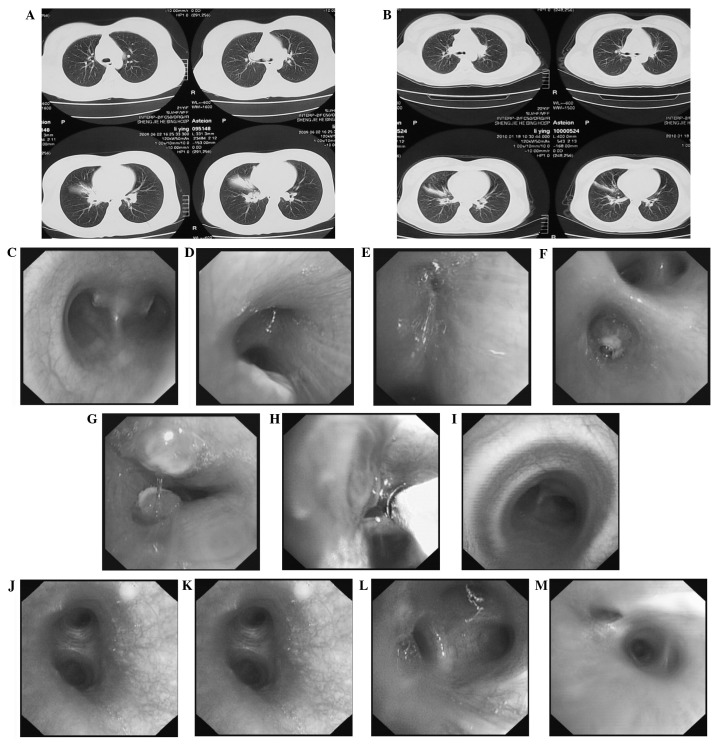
Case 1. (A). Computed tomography (CT) imaging prior to the cryotherapy procedure (7/3/2009); (B) CT imaging following the cryotherapy procedure (1/10/2010). Bronchoscopic images prior to treatment (7/27/2009) in the (C) carina; (D) left main bronchus; (E) upper lobe of the right lung; (F) middle and lower lobes of the right lung; (G) lower lobe of the left lung and (H) during cryotherapy. Bronchoscopic images following treatment (4/6/2010) in the (I) carina; (J) left main bronchus; (K) upper lobe of the right lung; (L) middle and lower lobes of the right lung and (M) lower lobe of the left lung.
